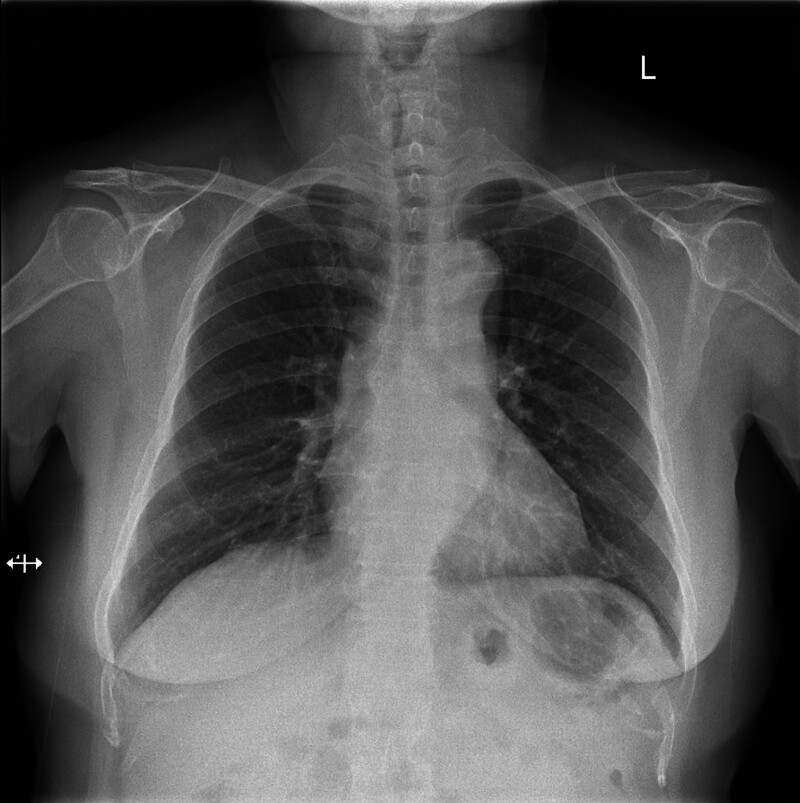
Chest radiograph shows that the enlarged goiter is causing the obvious airway compression and has pushed the airway to the right side.
